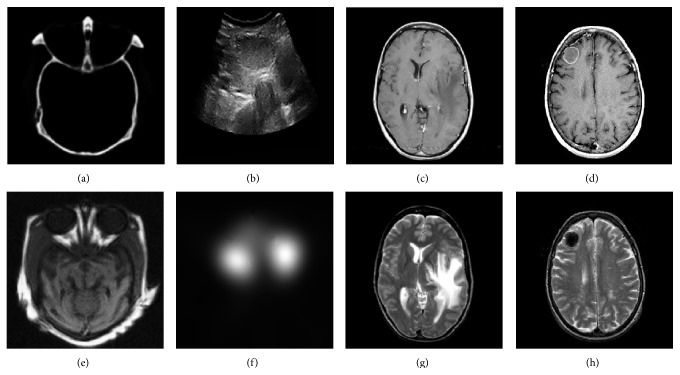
Different medical images to fuse. (a and e) show CT and MRI images of brain. (b and f) show B ultrasound and SPECT images of thyroid tumor. (c and g) show CT and MRI images of several focal lesions. (d and h) show T1-MRI and T2-MRI images that involved the lesion in the frontal lobe.
